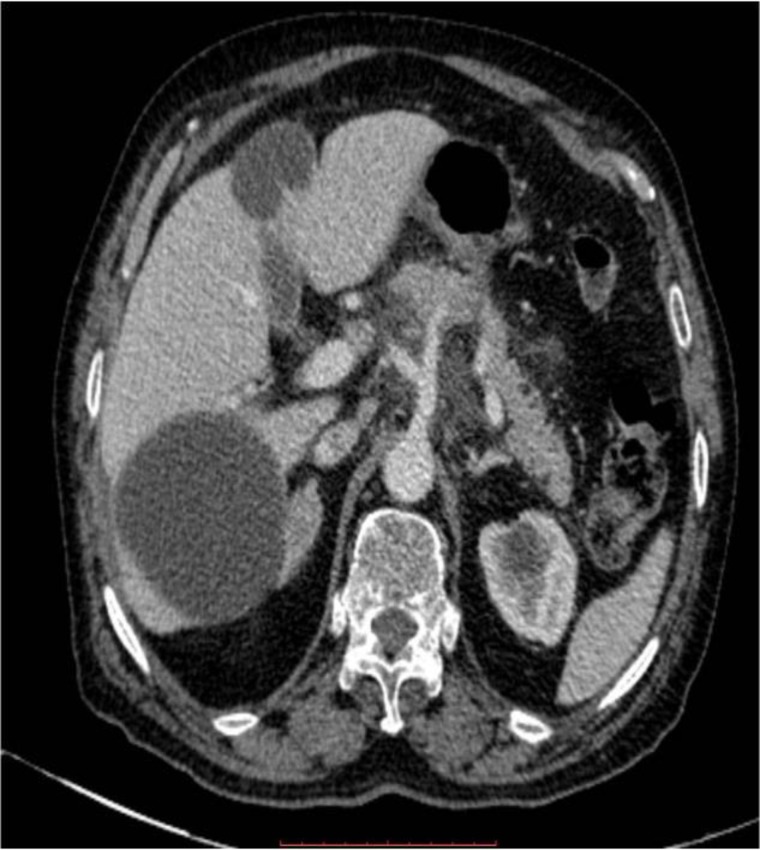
Axial contrast enhanced computed tomography reveals two round well defined homogenous hypodense lesions with thin imperceptible walls in segment V and VI of liver. Mild peripancreatic fat stranding is also present
